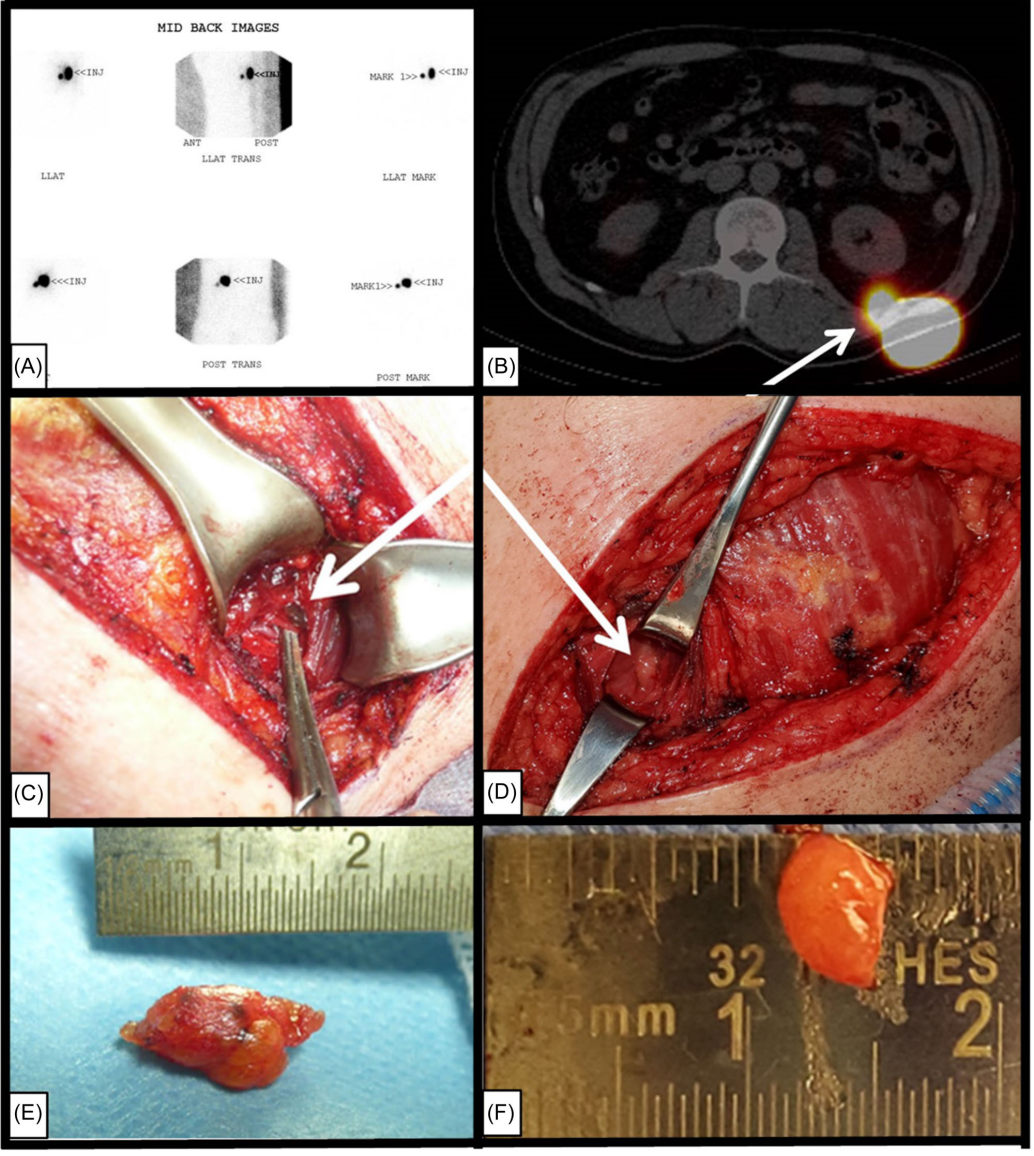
Pre, peri, and intraoperative imaging for patients S2 (A, C, E) and S5 (B, D, F). (A) lymphoscintigraphy showing a focus of activity noted lateral to the surgical site in the mid to lower back. (B) SPECT/CT revealing contiguous intense tracer activity immediately inferior to the left last rib. Intraoperative image of costal SN (arrow), inferior to the 12th rib (C, D), and intraoperative image of subcostal SN ex vivo (E, F). Melanoma metastasis was found in the subcostal SN shown in (C, E). SN, sentinel node; SPECT/CT, single‐photon emission computed tomography.
